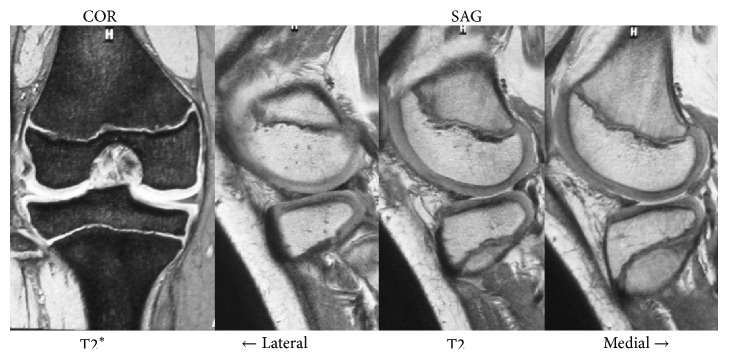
Magnetic resonance imaging (MRI), showing a defect in part of the load-bearing surface of the tibial lateral condyle articular cartilage.
